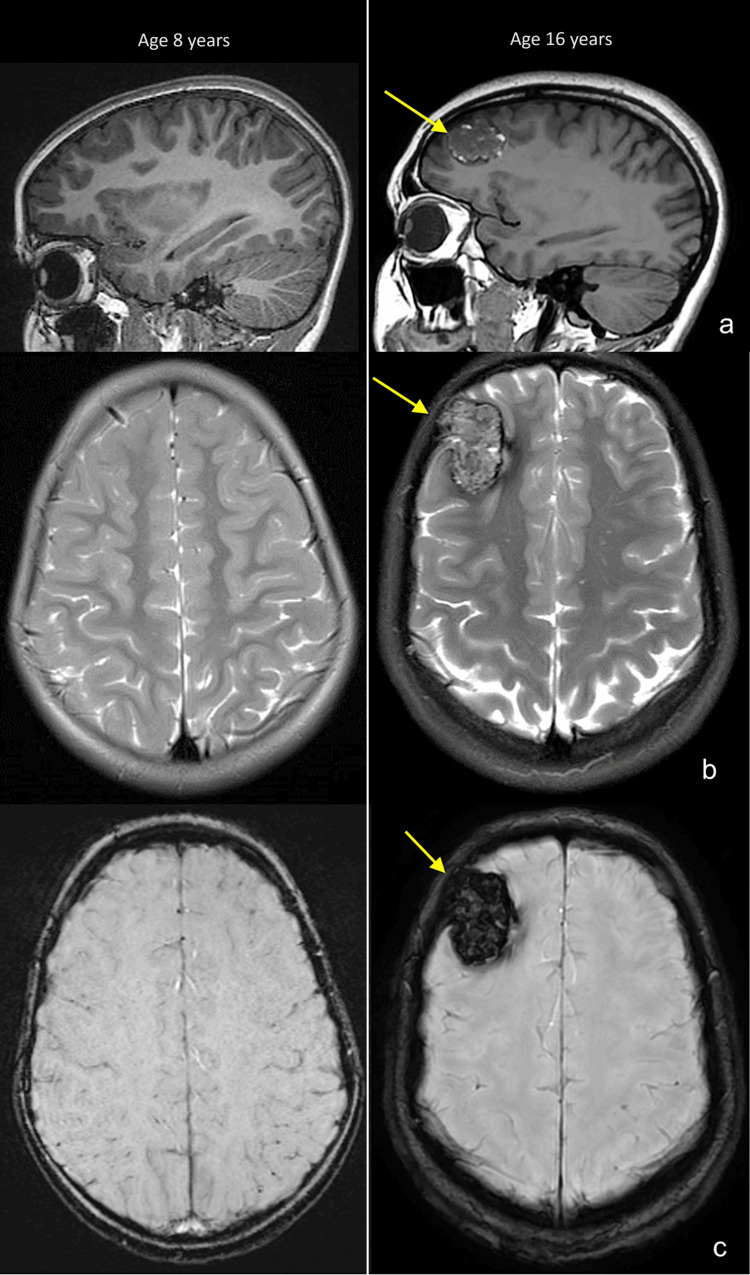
Sagittal T1 (a), axial T2 (b), and axial susceptibility-weighted images (c) from MRI scans of the patient at age eight years (left) and 16 years (right) demonstrate interval development of a new, large right frontal lobe cavernoma (arrows). MRI, magnetic resonance imaging
